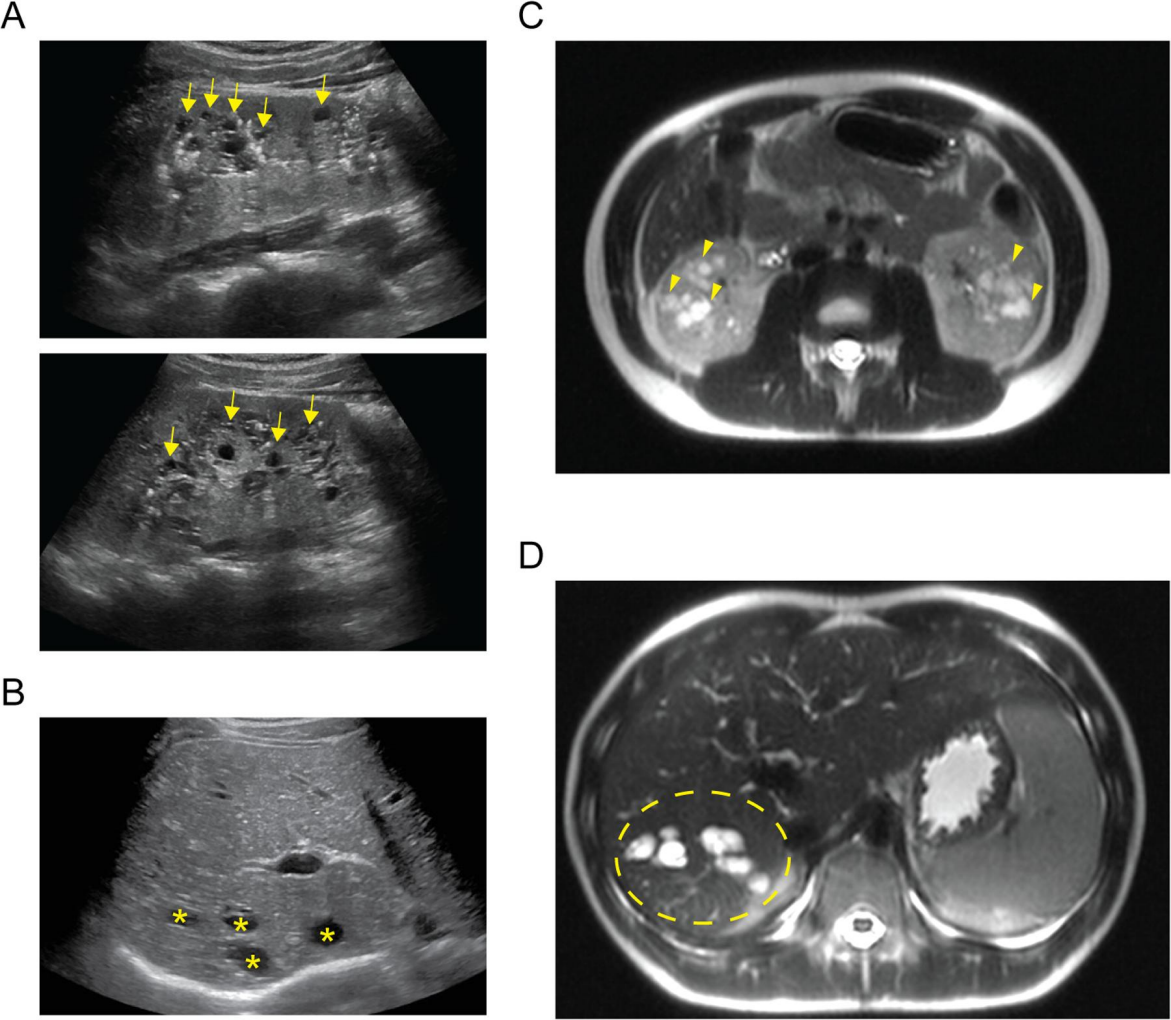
Imaging of cystic organ changes. (A) Sagittal ultrasound images of right (upper) and left (lower) kidneys demonstrating diffuse cystic changes predominantly affecting the medullary parenchyma (examples indicated with yellow arrows). (B) Transverse ultrasound image of the liver demonstrating cystic spaces of varying size affecting the hepatic parenchyma (examples indicated with yellow asterisks). (C) Axial T2-weighted MRI cross section demonstrating extensive kidney cystic changes bilaterally (examples indicated with yellow arrowheads). (D) Axial T2-weighted MRI cross section demonstrating extensive cystic changes within the right hepatic lobe (affected area within area demarcated by yellow dashed ellipse).
